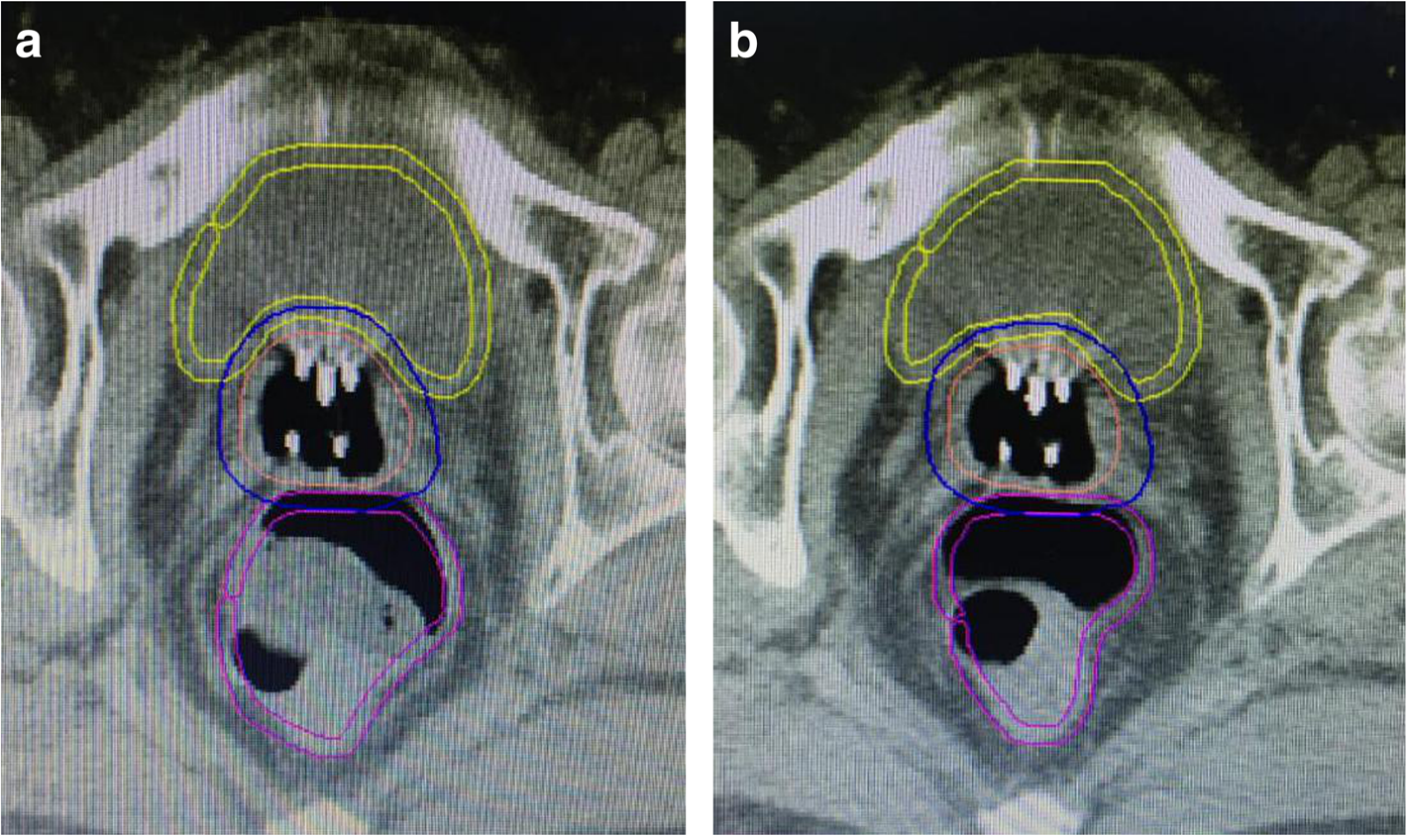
The CT images of a patient with KUSHEN Ningjiaos before insertion (a) and after insertion (b)
